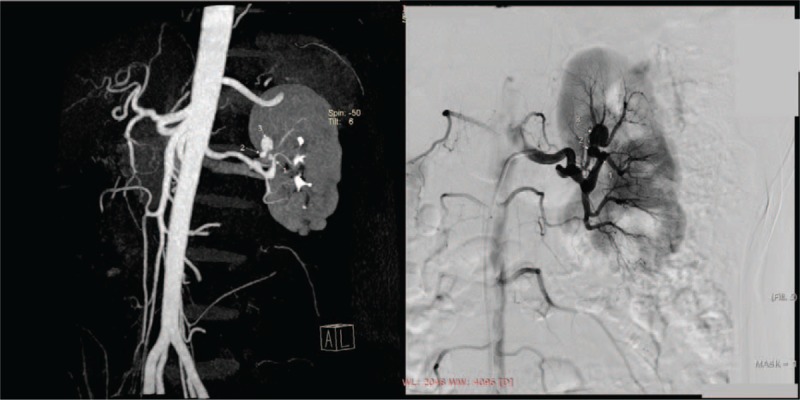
Computed tomography (2A) and angiography (2B) displayed three intrarenal aneurysms of the left kidney.
